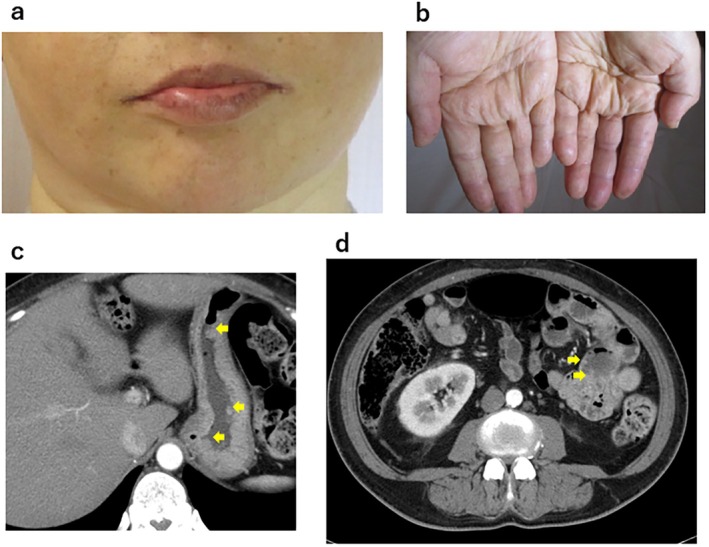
Findings pointing to Peutz–Jeghers syndrome. (a, b) Mucocutaneous pigmentation on the lips (a) and fingers (b). (c, d) CT finding of multiple gastric polyps (c) and small intestine polyps (d). Arrows indicate polyps.
